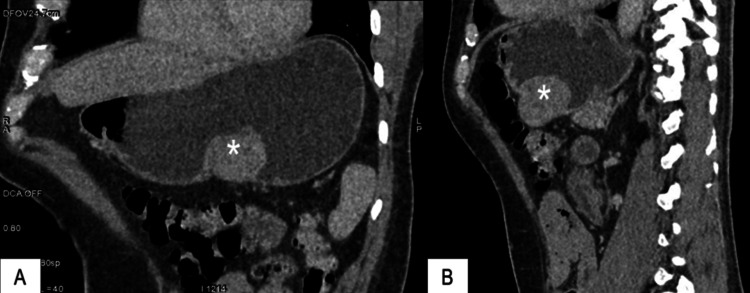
CT scan of the abdomen showing the lesion in coronal and sagittal planes (A) Coronal oblique reconstruction CT and (B) oblique sagittal reconstruction CT. CT: Computed tomography.
